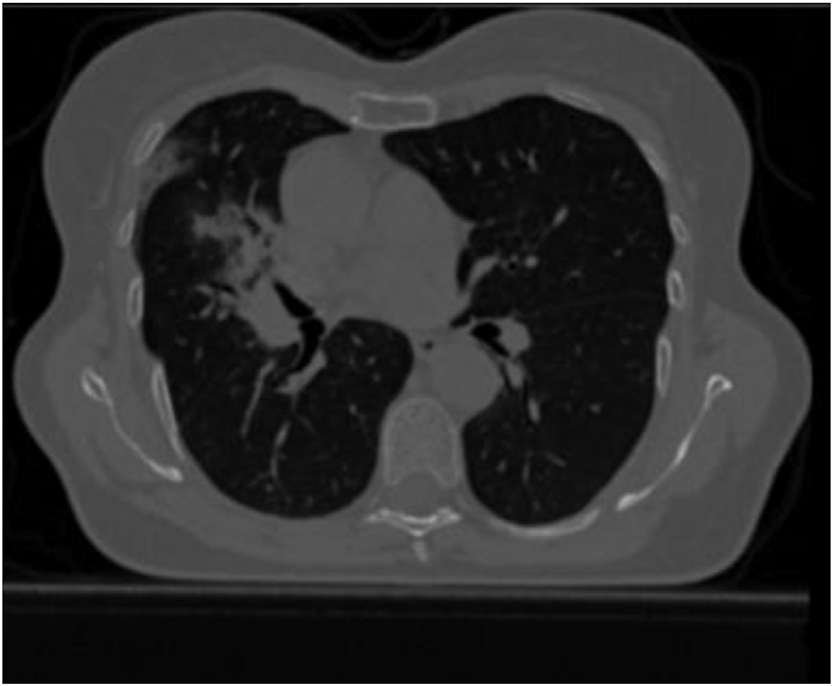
Chest CT slice of PCR positive patient (B2) showing GGO in right upper lobe and consolidation opacity in the right middle lobes.
